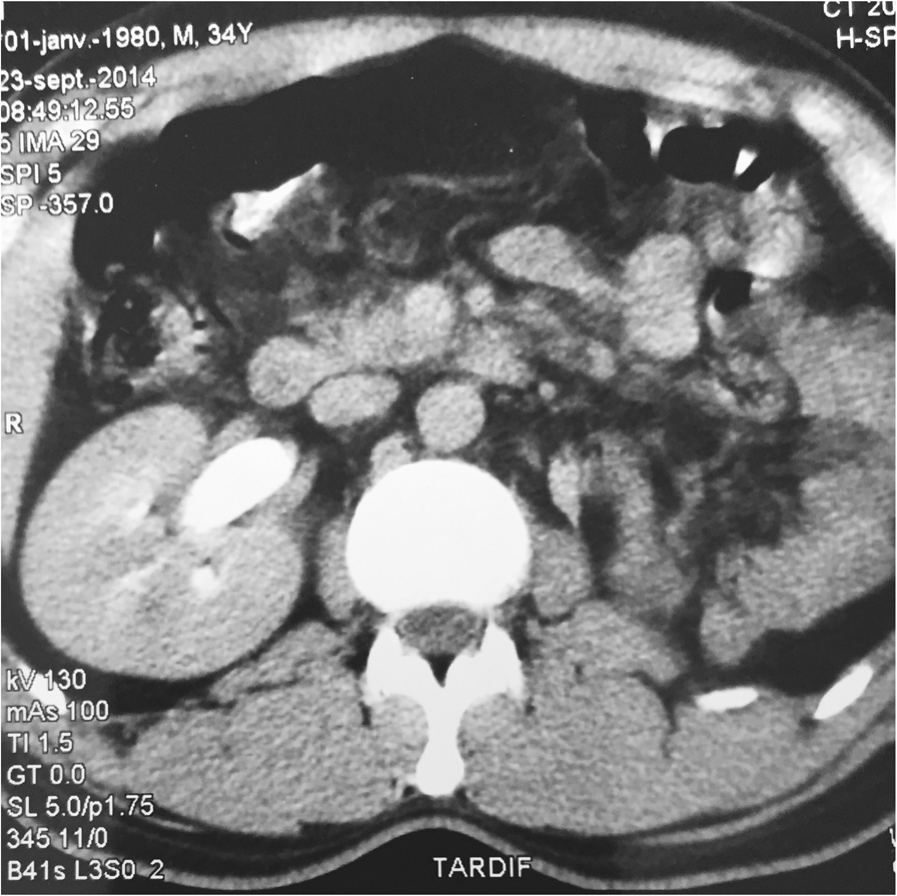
CT image showing a single right kidney
